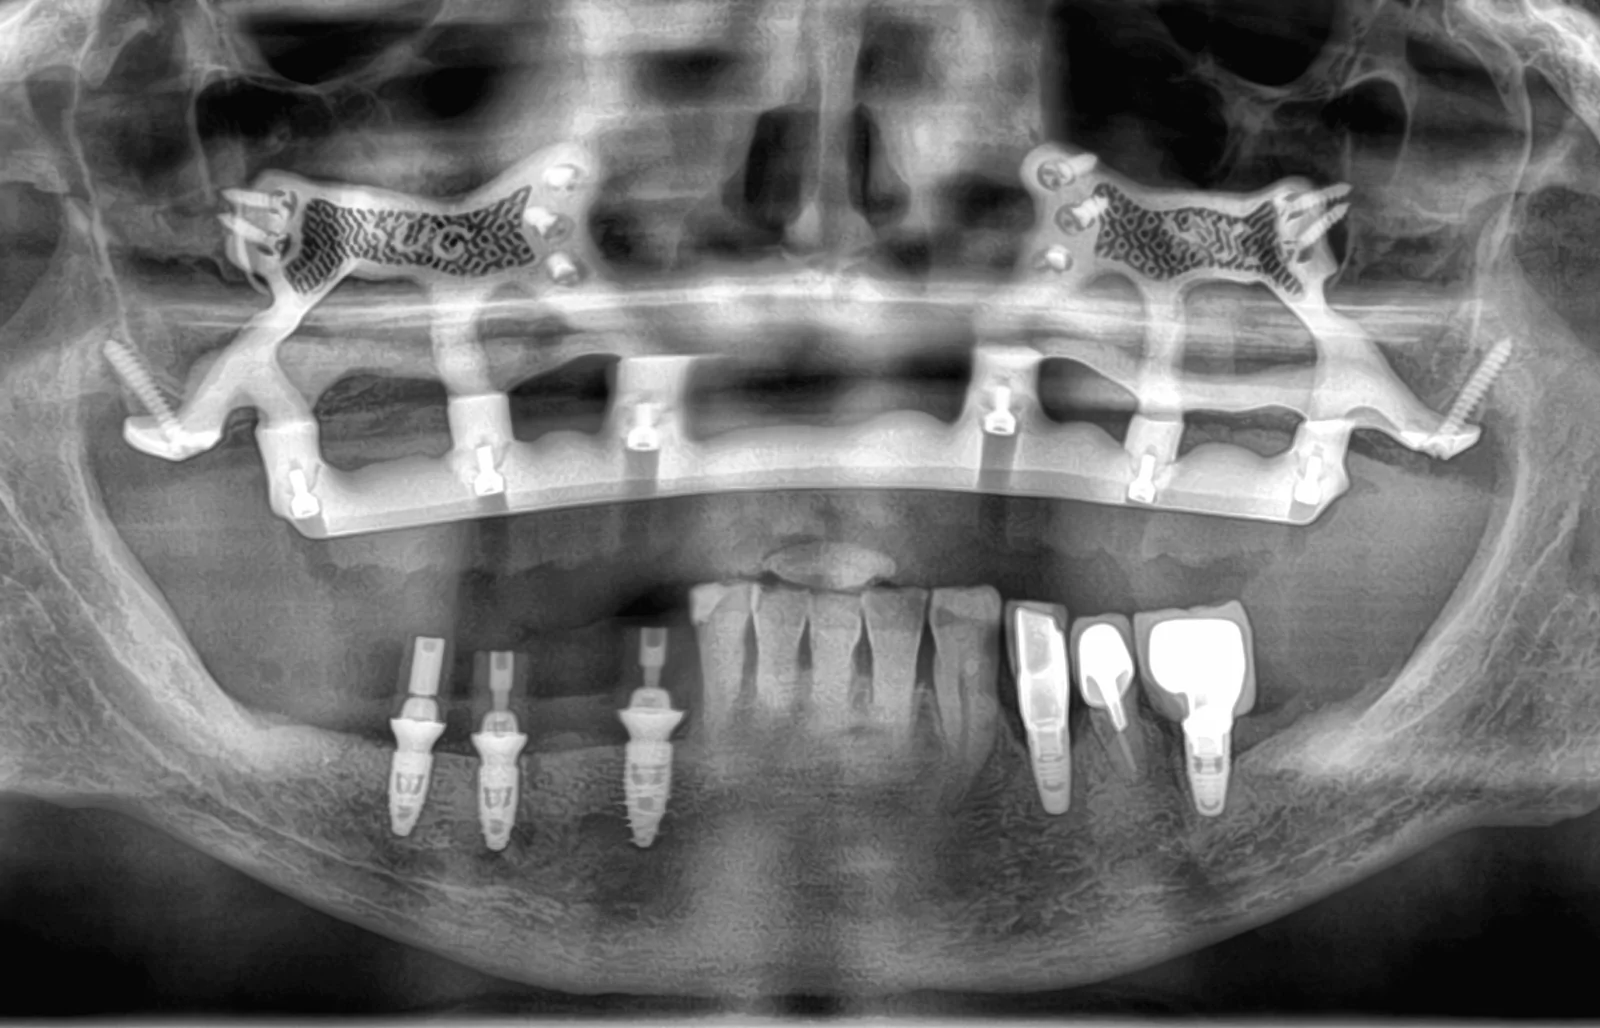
Postoperative panoramic X-ray imaging.
